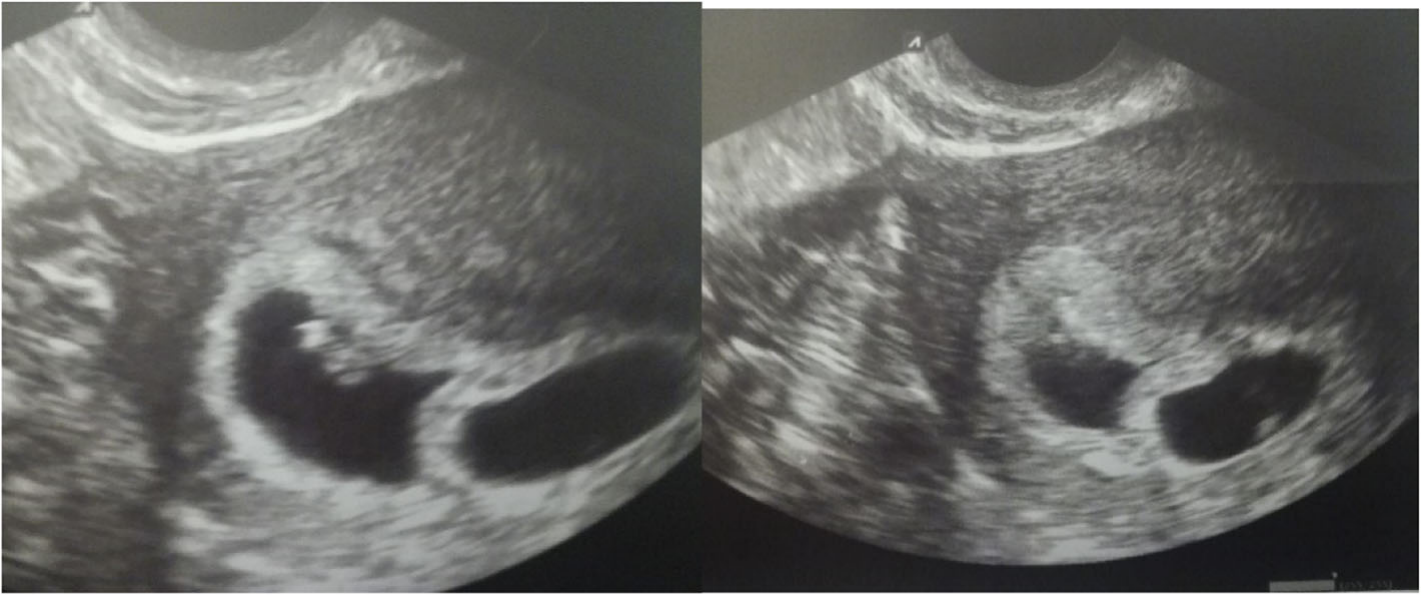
Ultrasound examination showing pregnancy confirmation
